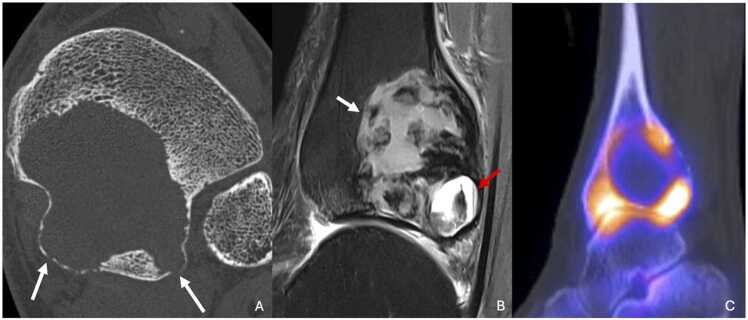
Giant cell tumor in a 59-year-old man with mechanically induced left ankle pain. A. CT image in the axial plane highlighting a large osteolytic lesion with multifocal cortical disruptions (white arrows). B. T2-weighted fat-suppressed MR image in the sagittal plane showing heterogenous signal with solid (white arrow) and cystic areas (red arrow). C. Bone scintigraphy in the sagittal plane highlighting “the donut sign” corresponding to peripheral hyperfixation.
